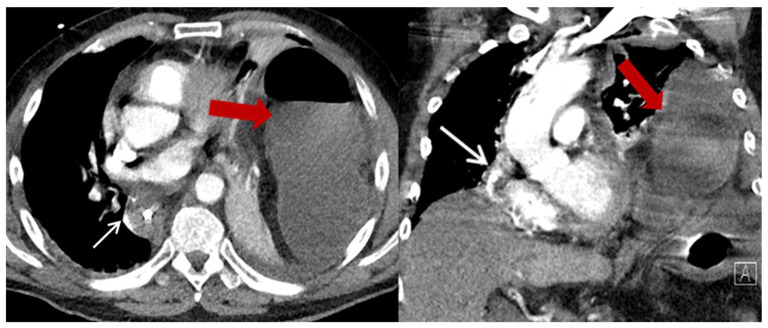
Exemplary CT-scan of an incarcerated hiatal hernia on the left thoracic side with a clear mediastinal shift to the right. Gastric conduit marked with thin white arrow; herniated colon marked with thick red arrow.
